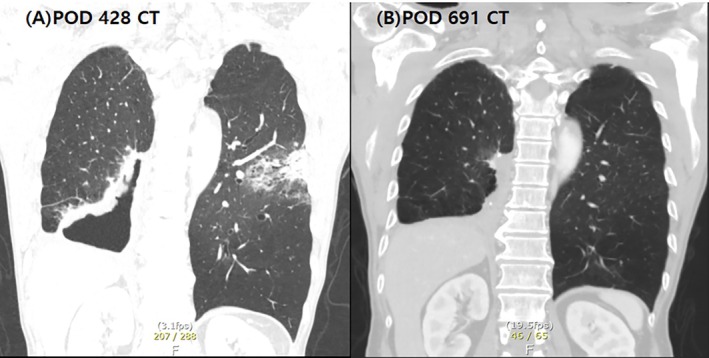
Case 1: Serial chest CT scans showing the course of the right lower hemithoracic cavity caused by
